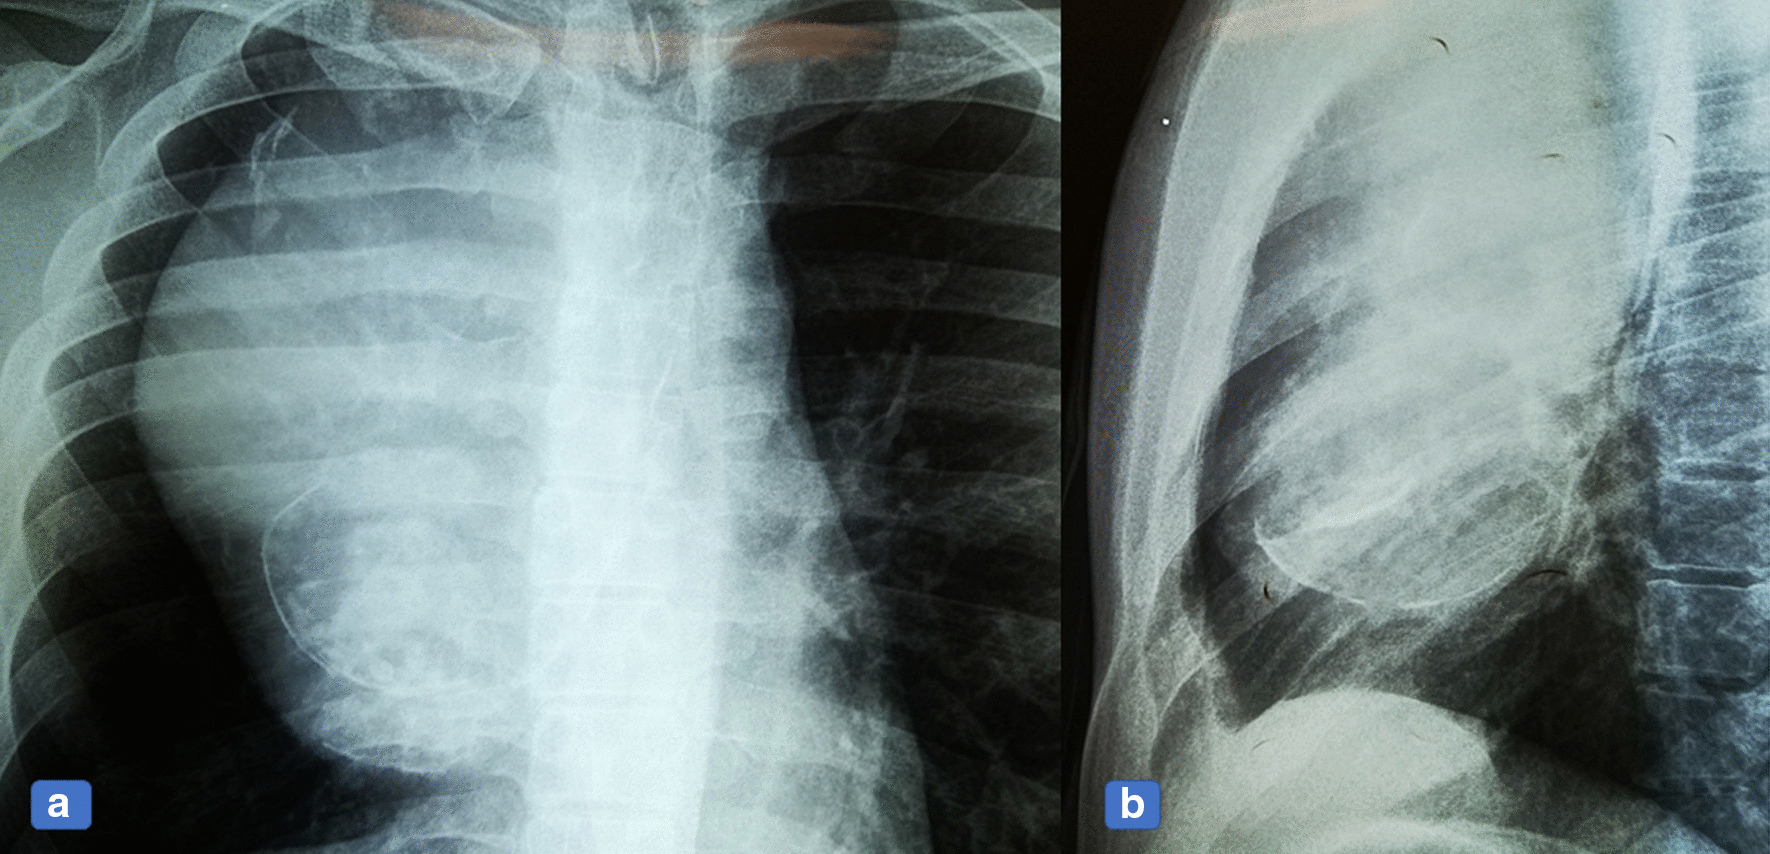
a Chest x-ray, Anterior Posterior (AP) view, showing a large, lobulated, soft tissue density mass with internal calcification. It forms an obtuse angle causing superior mediastinal widening, more on the right side. b Lateral chest x-ray shows obliteration of the retrosternal space.
